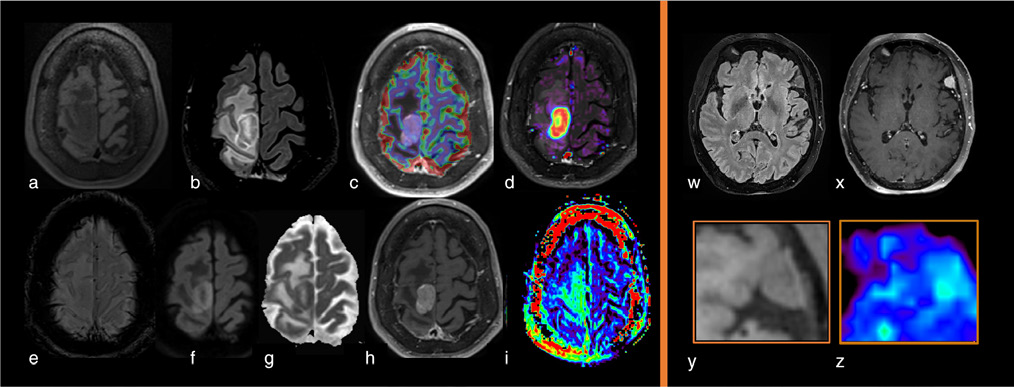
Example of a primary central nervous system lymphoma (A – I) and of a meningioma (W – Z), both displaying high signal intensity on F.S. APTw imaging (see I for lymphoma and Z for meningioma). (a) 3D T1w SE. (b) 3D FLAIR. (c) rCBV map after leakage correction superposed to T1w injected sequence. (d) K2 cartography (derived from DSC perfusion) superposed to FLAIR. (e) SWI. (f) DWI. (g) ADC map. (h) 3D T1w injected sequence. (i) APTw map. Of note, APTw hyperintensity goes beyond contrast enhancement and covers the quasi-totality of the FLAIR contrast. (w) 3D FLAIR. (x) axial 3D T1w injected sequence. (y) zoom on the extra-axial lesion on T1w without contrast injection. (z) zoom on APTw map. 3D, three-dimensional; ADC, apparent diffusion coefficient; APTw, amide proton transfer-weighted; FLAIR, fluid attenuated inversion recovery; rCBV, relative cerebral brain volume.
